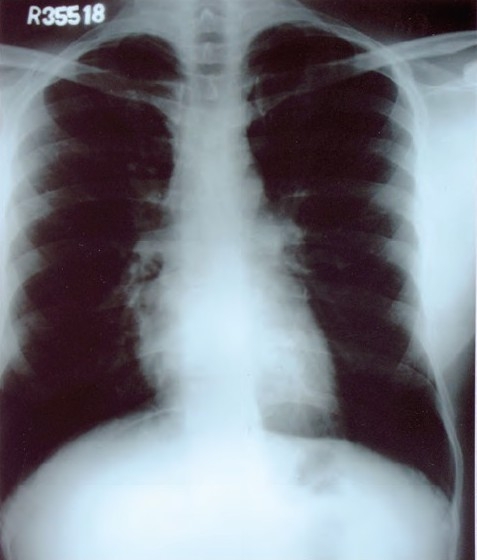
Normal chest radiograph film without any mediastinal lymphadenopathy.
